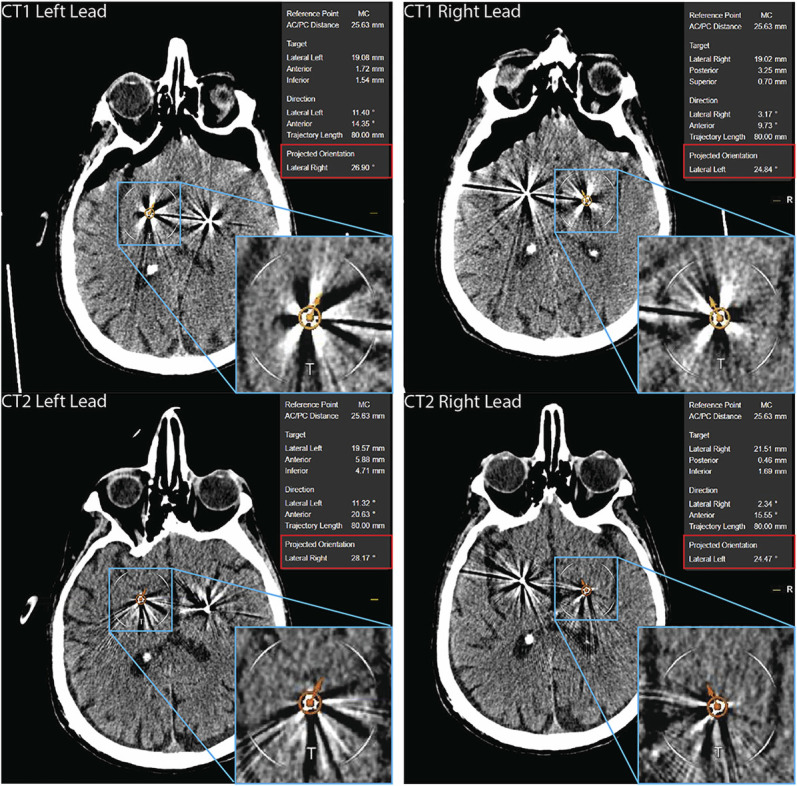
Orientation angles found on CT1 and CT2 based on the DiODe algorithm. Automatic lead detection using the Brainlab software's built-in DiODe algorithm provides an estimation of the lead orientation for the anterior commissure/posterior commissure line. Orientation of the left lead in CT1 (yellow arrows) and CT2 (red arrows) is displayed on the left panels. Orientation of the right lead in CT1 and CT2 is displayed on the right panels. Embedded images highlight the estimated orientation of the leads. “Projected orientation” (in the red box) is the value of the estimation. Lead rotation is calculated by subtracting the projected orientation angles found on CT1 and CT2. CT, computed tomography; DiODe, directional orientational detection.
